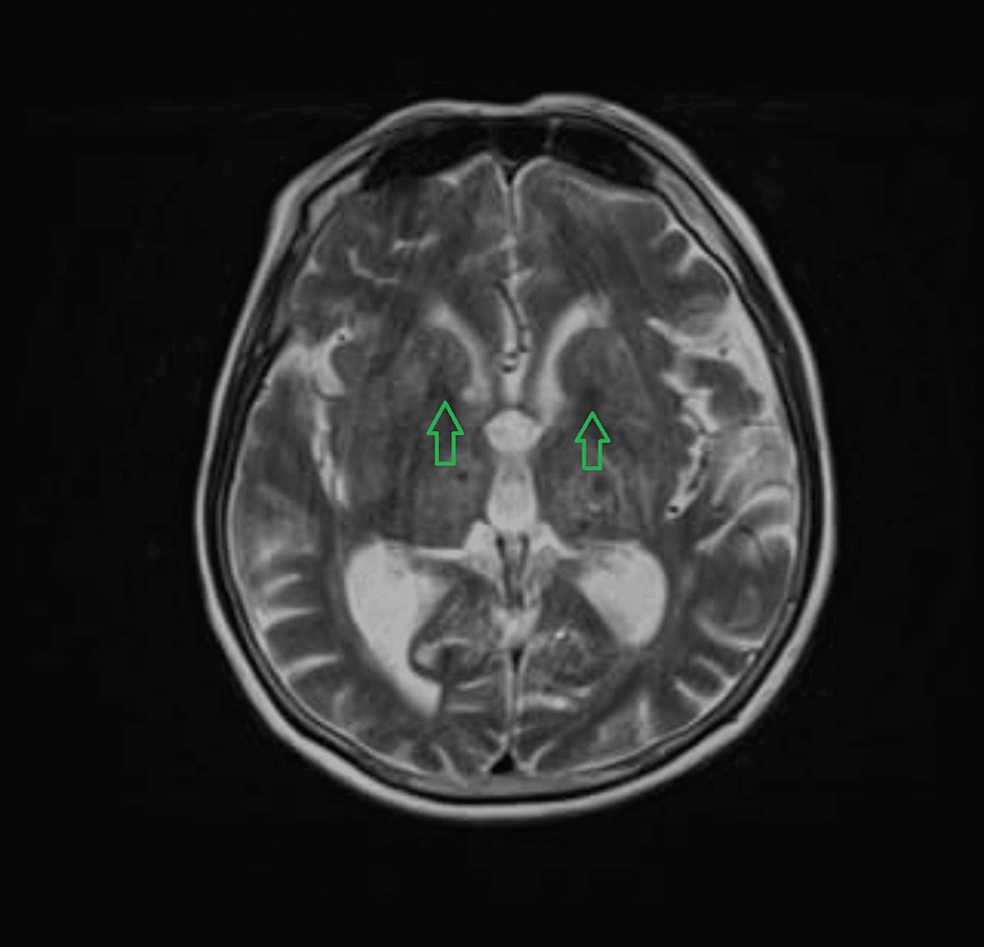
MRI brain indicating recent small haemorrhages in the bilateral basal ganglia region MRI: magnetic resonance imaging
